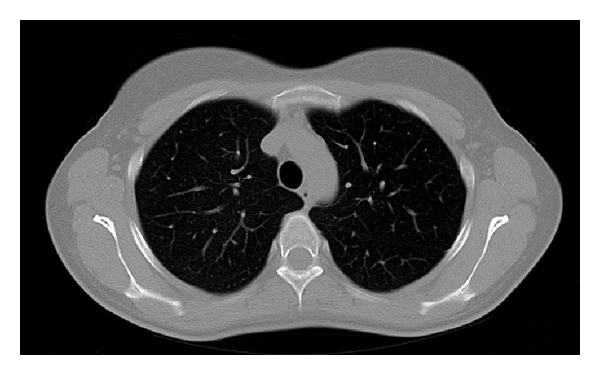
CT-scan of the same region depicted in Figure 3, three months later, showing complete remission of the right component of the bilateral pneumonia.
